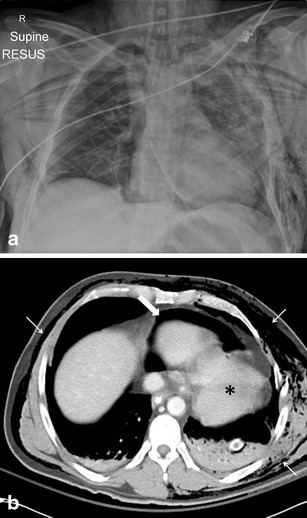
A 45-year-old male involved in a road traffic accident. a The chest radiograph identified the presence of pneumopericardium most evident adjacent to the right heart border with cardiac displacement. There are multiple injuries but note the gross surgical emphysema that limited the FAST assessment.b On the selected axial CT image on soft tissue window settings, there is extensive surgical emphysema (*thin white arrows*), pneumopericardium (*thick white arrow*) and note the displacement of the heart posteriorly into the left hemithorax (*black asterisk*). This patient had sustained a left-sided pericardial tear with cardiac herniation that was surgically repaired. (a Reprinted with permission from [18])
